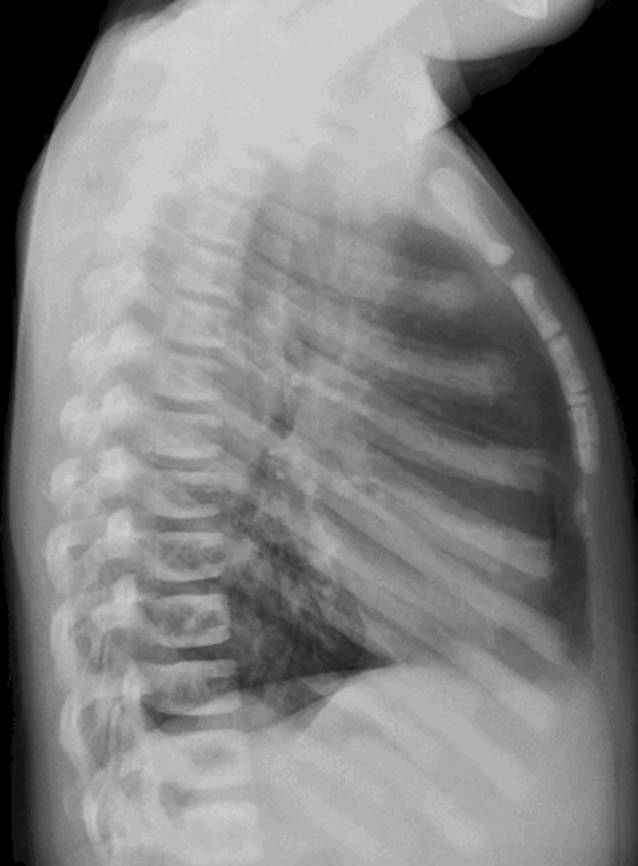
Lateral chest radiograph showing generalized increase in bone density with typical sclerosis of vertebral endplates resulting in “sandwich vertebrae” appearance and widening of the ribs at the costochondral junction
